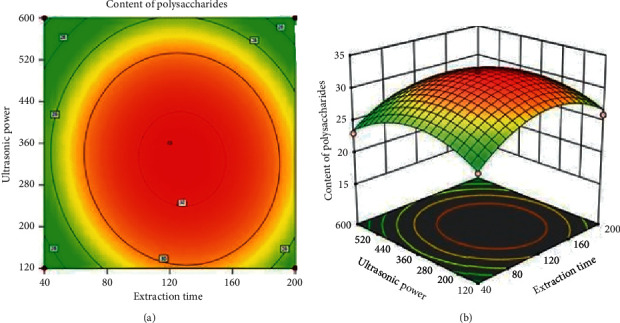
Simultaneous effect of extraction time and ultrasonic power on the content of polysaccharides.
